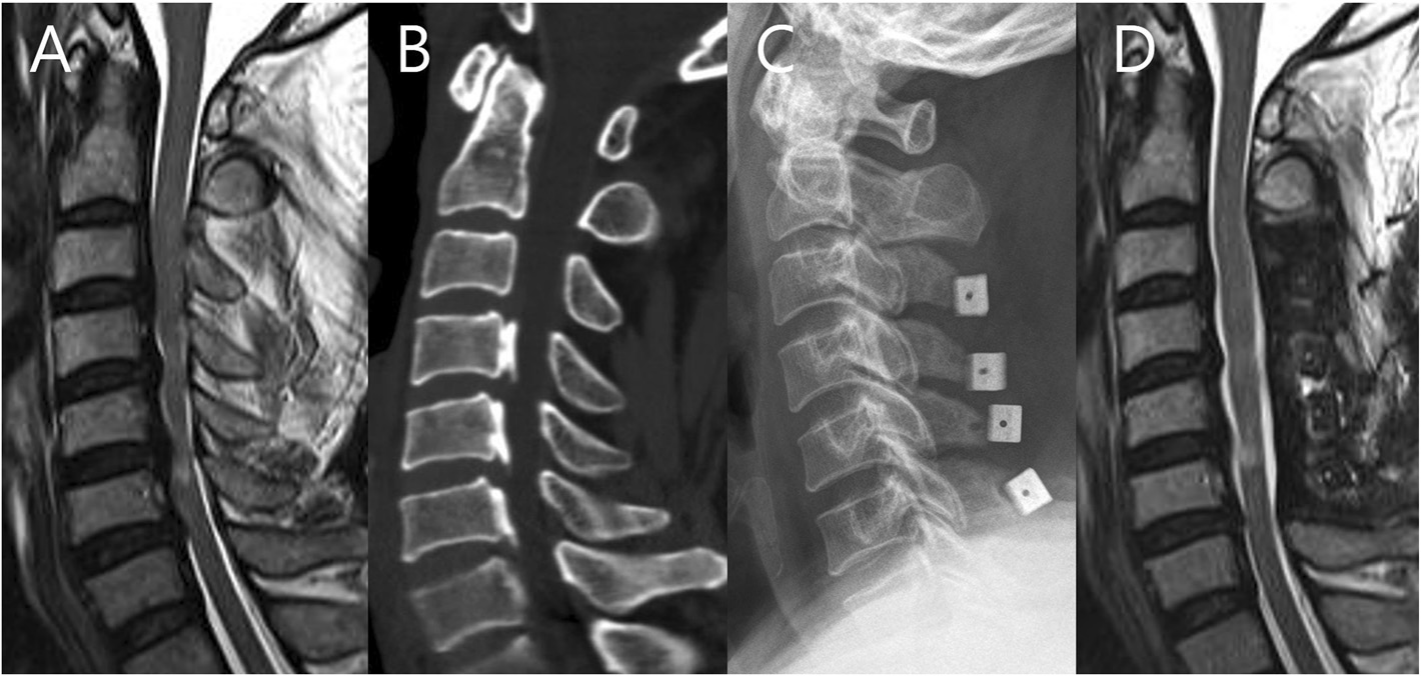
A patient with intramedullary signal intensity grade 2. a Preoperative T2-weighted MRI results for a 54-year-old male patient with spinal cord compression at C3-4-5-6-7. Intramedullary high signal intensity (SI), grade 2, was visible at the region of the marked compressed cord. Initial ASIA motor score was 48. b Cervical 3D CT showed mixed type ossification of the posterior longitudinal ligament (OPLL). Spinal canal stenosis (SAC) was 5.9 mm. c The patient underwent cervical laminoplasty at C3-4-5-6. d MRI of the patient from B 12 months after cervical laminoplasty with successful decompression of the spinal cord. The patient’s ASIA motor score was 72. The recovery rate was 46.2 %
